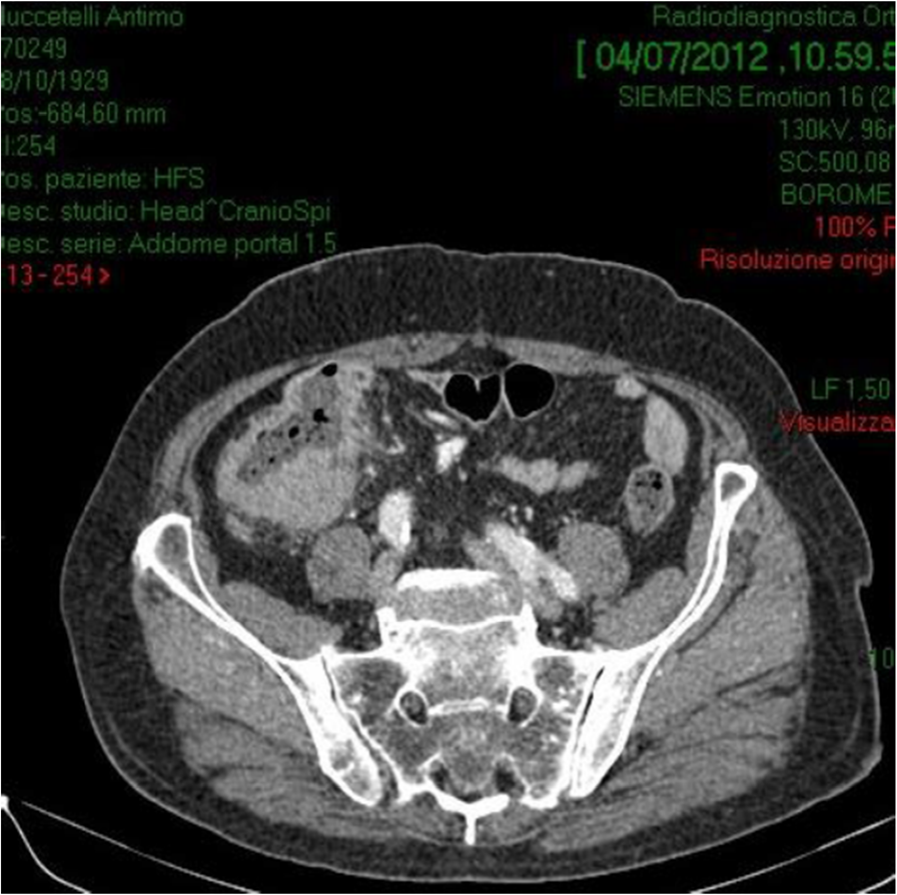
Abdominal computed tomography (CT) with intravenous contrast detected a ulcerated massat the proximal ascending right colon, with associated adenopathy of the ileo-colic pedicle.
